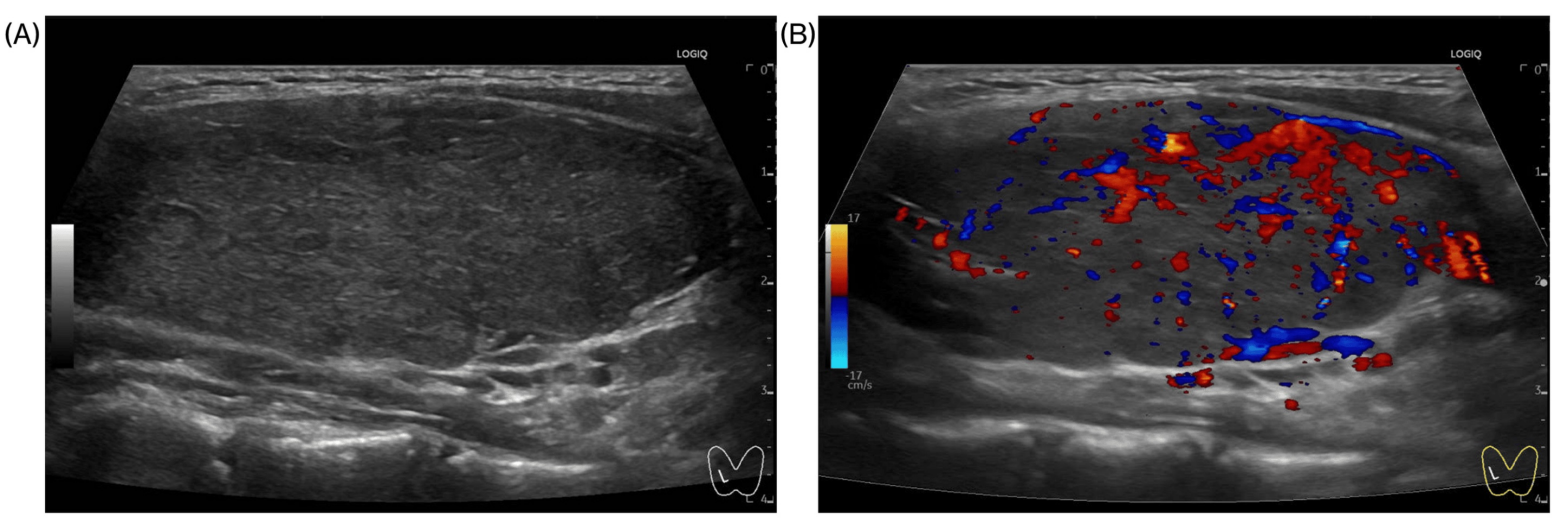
Ultrasonographic images of Graves' disease Longitudinal ultrasonographic images of the thyroid gland of a 10-year-old girl with Graves' disease during GnRH agonist therapy. (A) B-mode ultrasonography revealed diffuse thyroid enlargement with homogeneous parenchymal echogenicity. (B) Color Doppler imaging demonstrates marked hypervascularity with a “thyroid inferno” pattern, a characteristic finding of Graves' disease. GnRH, gonadotropin-releasing hormone
